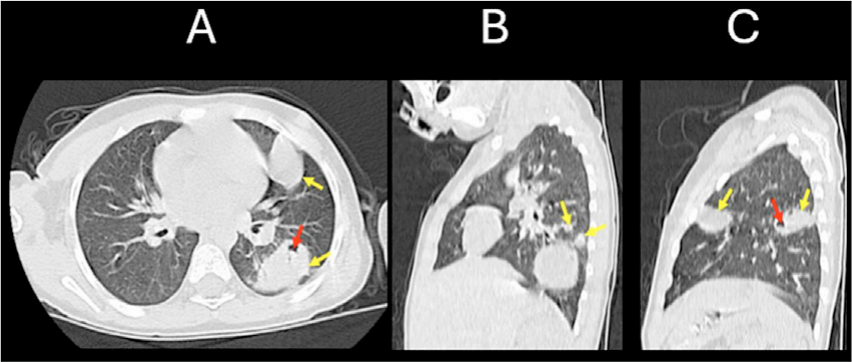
CT scan of the chest in lung window settings. Multiplanar views including axial (A), right lung sagittal (B), and left lung sagittal (C) images demonstrate multiple well-defined, thin-walled cystic lesions distributed across both lungs (yellow arrows). The left lung contains an excavated cyst with internal air-fluid level (red arrow), indicative of partial cyst rupture or bronchial communication.
